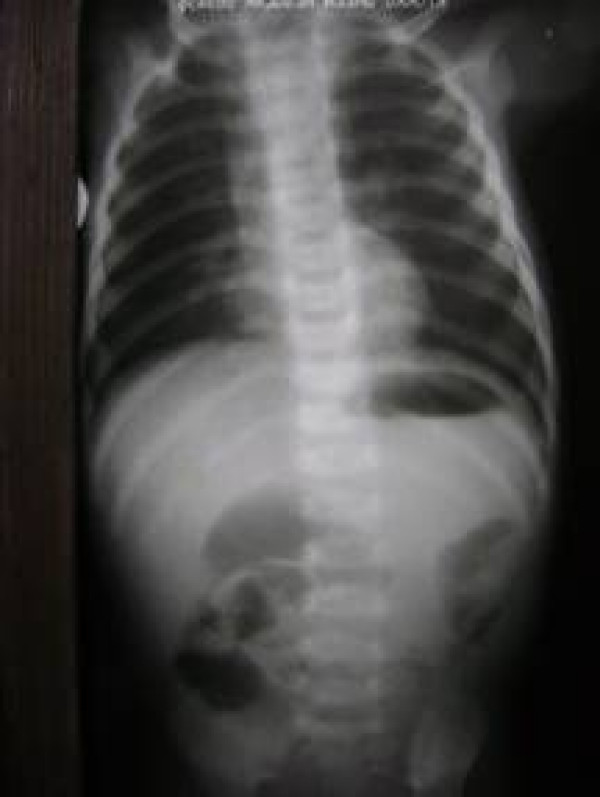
plain x-ray abdomen shows multiple fluid level.
